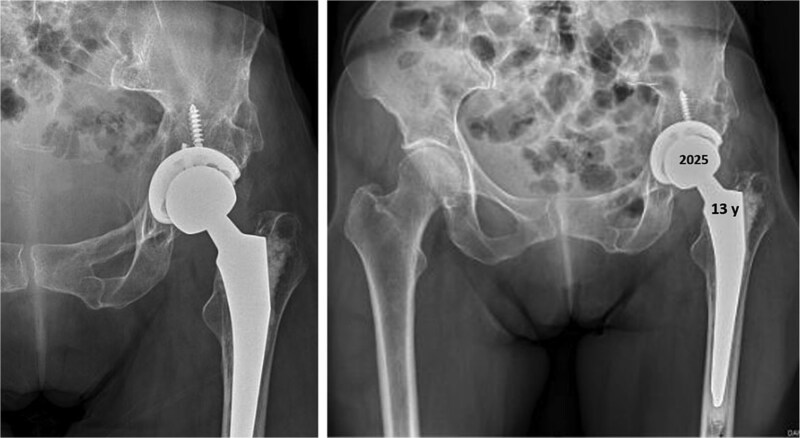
Postoperative anteroposterior radiographs of the pelvis 13 years after the THA, showing mechanical stability of the prosthesis with no evidence of loosening. No signs of osteoarthritis are present in the right hip (Tönnis Grade 0).
